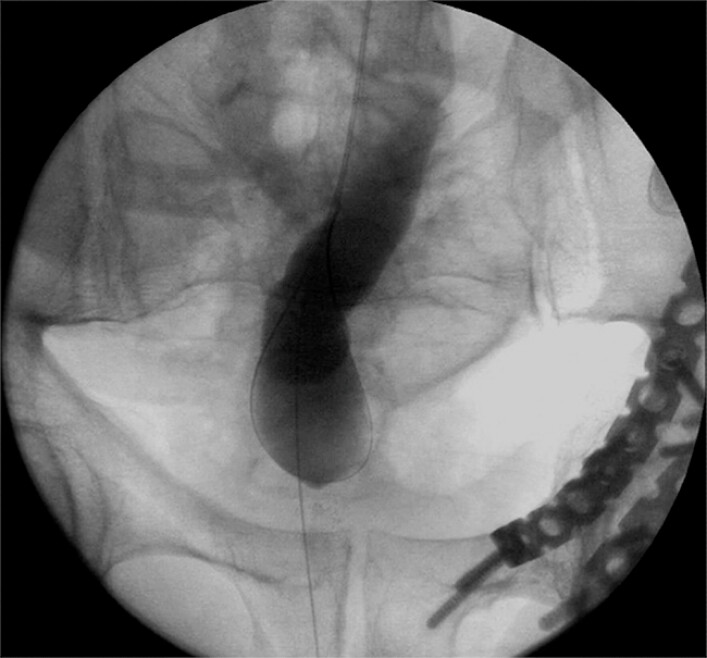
X-ray fluoroscopy showed the mixed solution of contrast medium and methylene blue in the intestinal cavity on the oral side of the obstruction.
